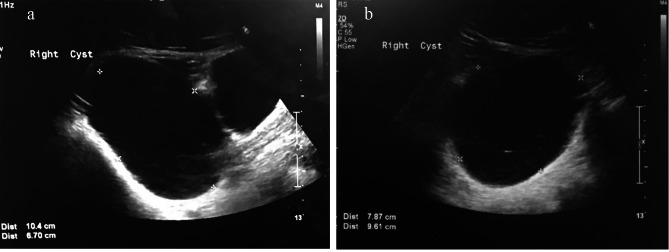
(A)(B) Abdominal sonographic scanning with size measurement of the mass
